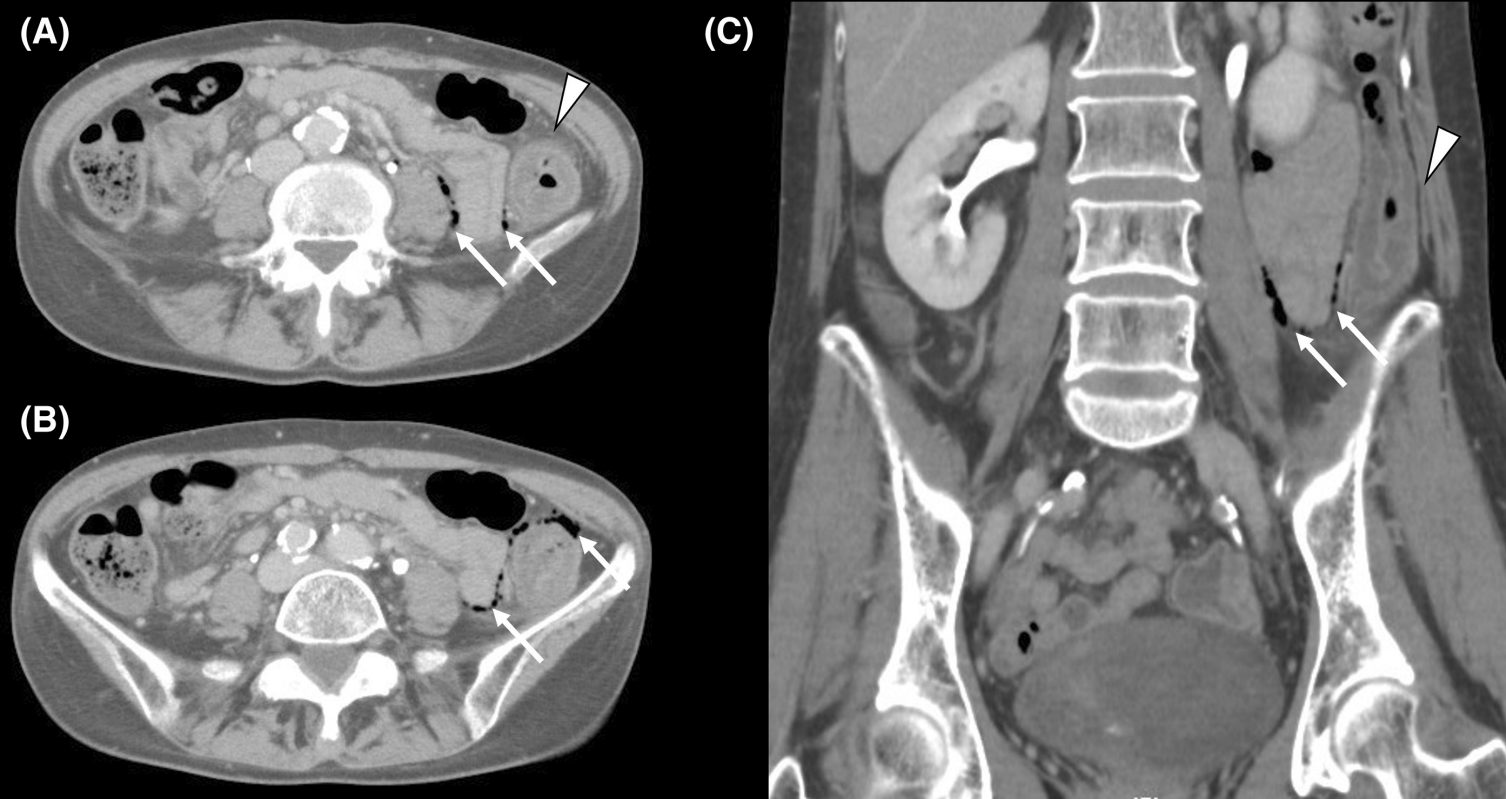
The initial CT scan reveals thickening of the wall of the descending colon (A, C arrowhead) and free air around the colon and in the retroperitoneum (B, Carrow). CT, computed tomography.
